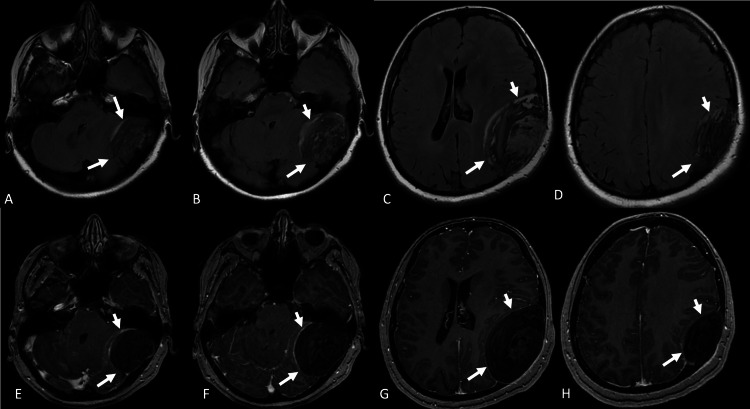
Preoperative axial T2 FLAIR (A-D) and post-contrast axial T1-weighted images (FSPGR BRAVO sequence) (E-H). White arrows point to the mass lesion. FLAIR, fluid-attenuated inversion recovery
